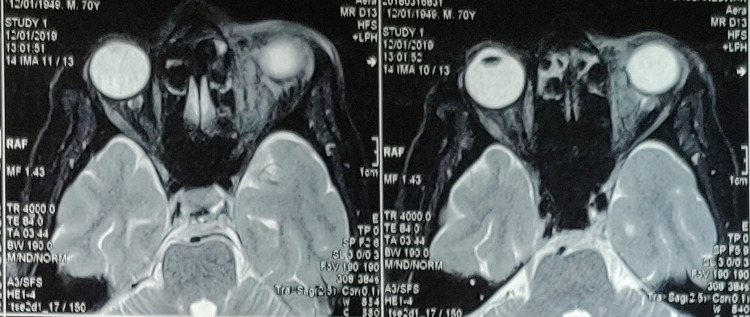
MRI orbital axial T2 image Hypointense lesion in left orbit involving the medial rectus and extending to postero-medial aspect of superior rectus abutting the lateral part of left optic nerve sheath
